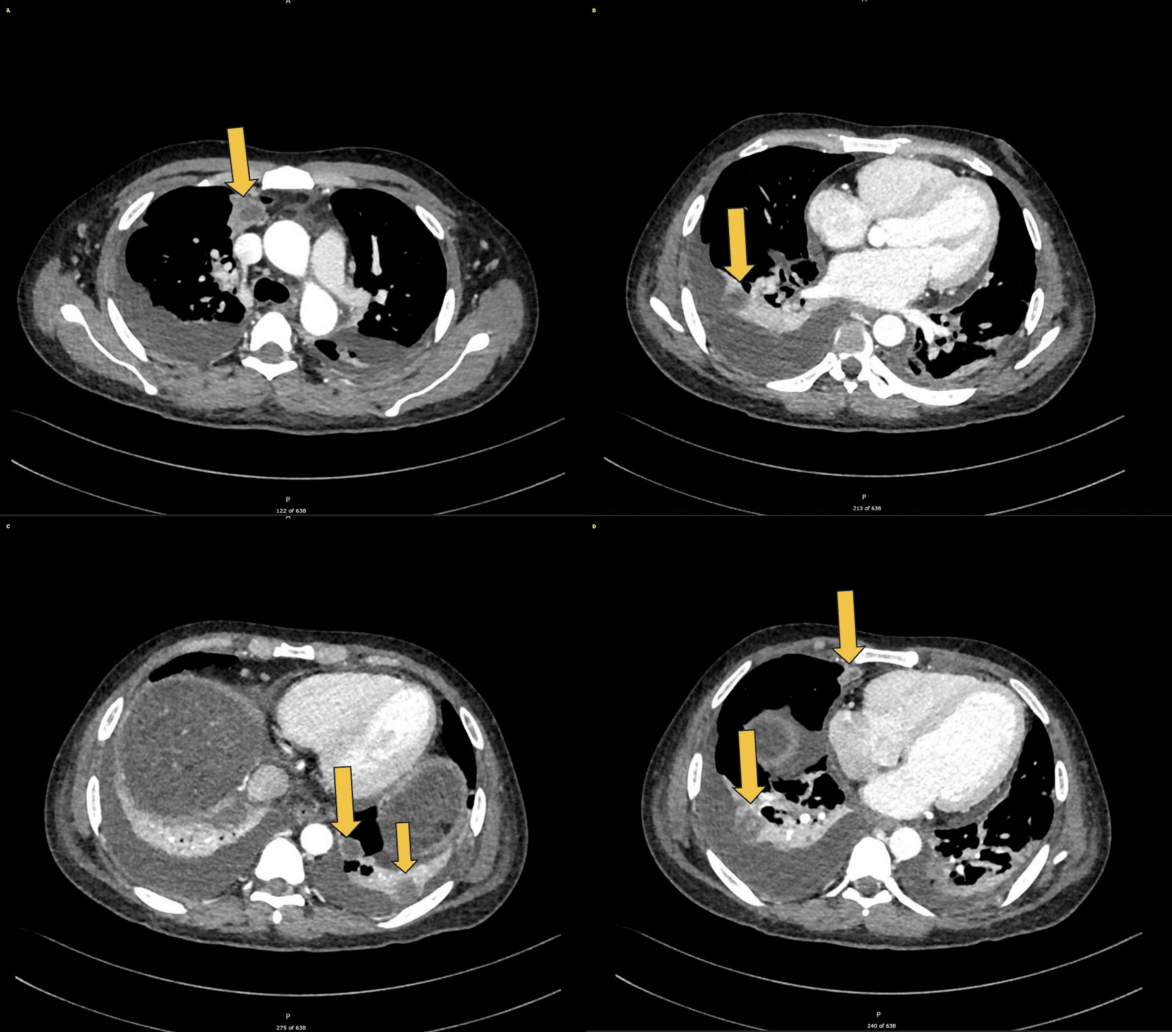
CT of the chest revealing multiple sections of different levels showing bilateral lung abscesses appearing as localized low-attenuation areas (yellow arrows) CT: computed tomography
